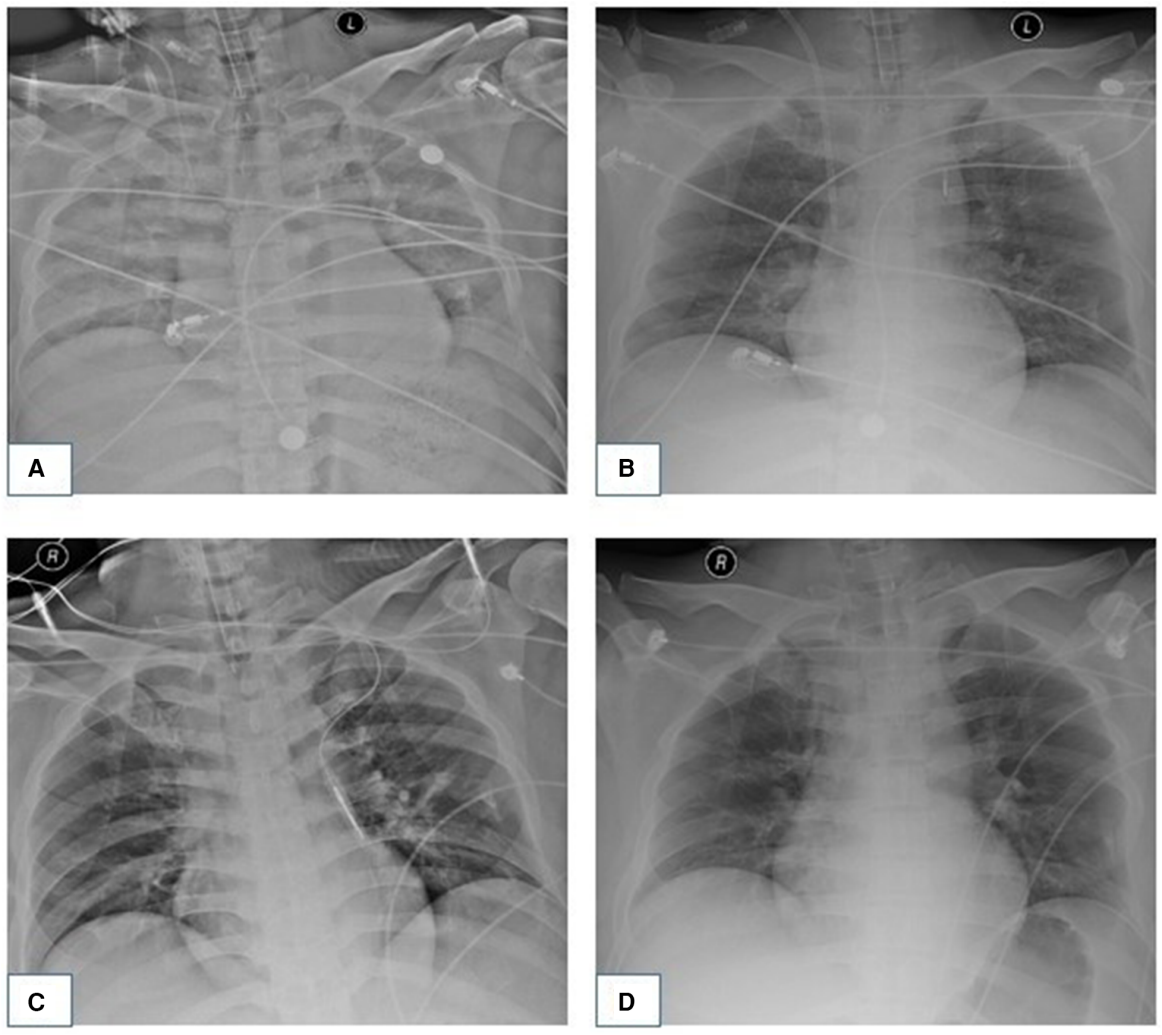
Dynamic changes of the patient's chest x-ray examination. (A) On August 18, 2021, chest radiograph showed that bilateral pneumonia and bilateral pulmonary exudative lesions were severe. (B) On August 21, 2021, double pneumonia and exudative lesions showed improvement compared with those in the last assessment. (C) On August 31, 2021, the patient's chest radiograph showed a significant improvement in double pneumonia. (D) The patient's pneumonia had been cured by September 6, 2021.
